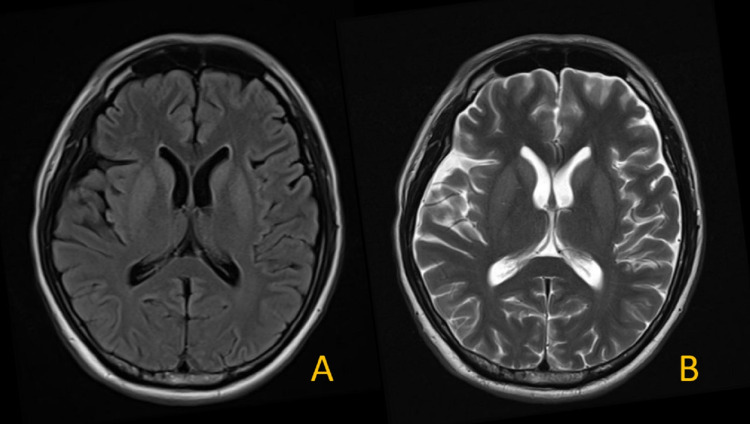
Brain MRI on day two of hospital admission. The axial FLAIR (A) and T2WI (B) images show no significant abnormalities. FLAIR: Fluid-attenuated inversion recovery; T2WI: T2 weighted image
